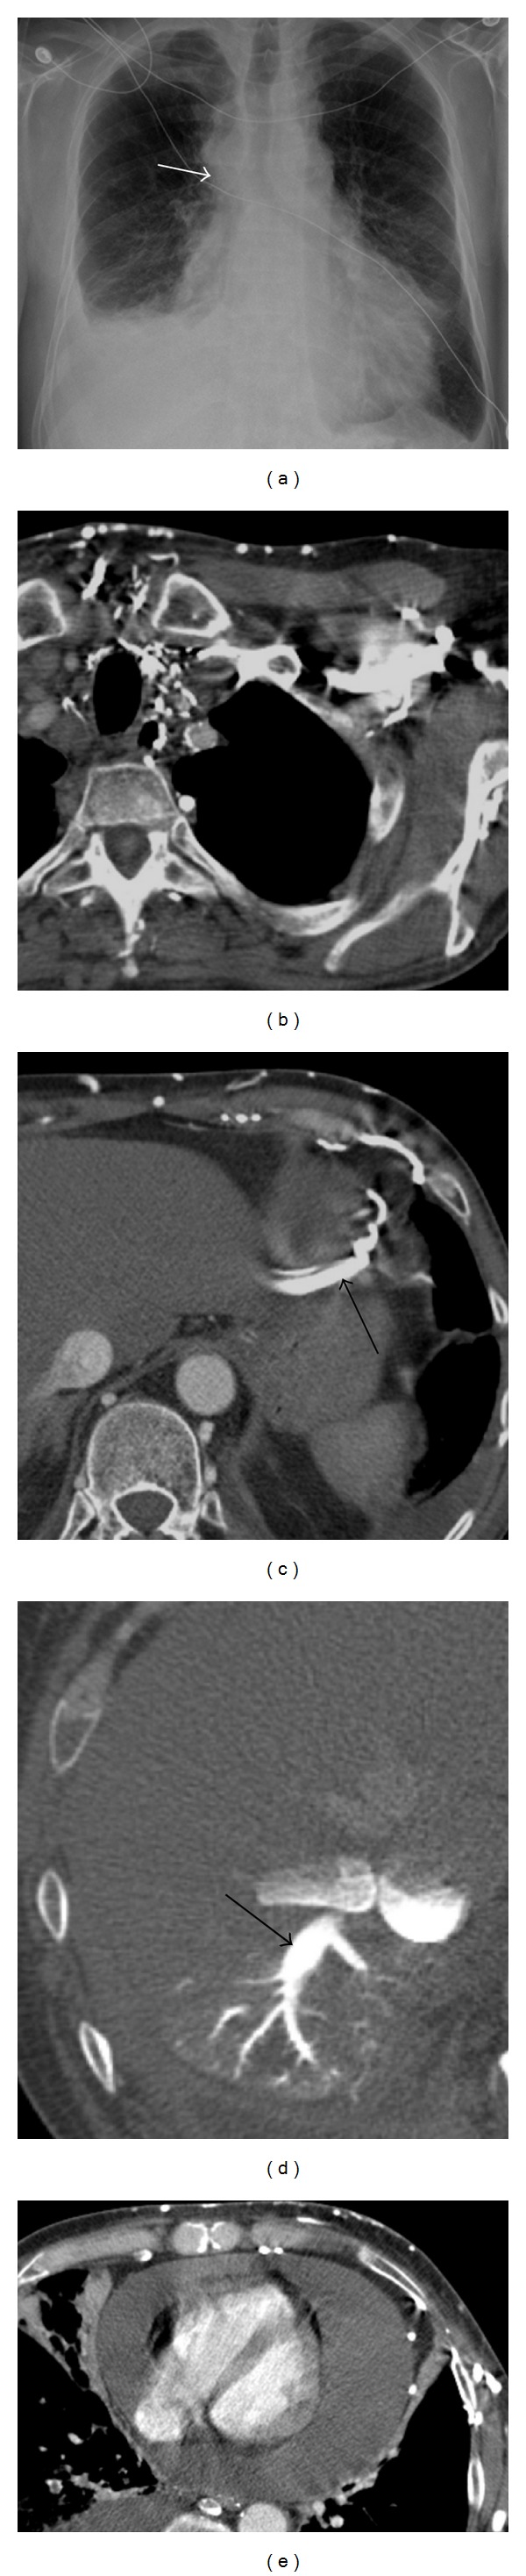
A 55-year-old previously healthy male with dyspnea. (a) Chest radiograph shows an enlarged cardiac silhouette, a right pleural effusion, and dilated azygos vein (arrow), (b) CECT demonstrates extensive venous collaterals around the heart, including prominent filling of (b) subcutaneous, (c) inferior phrenic, and (d) hepatic veins. (e) CECT shows a large pericardial effusion. The interventricular septum is flattened. The constellation of effusion, flat septum, and impaired venous return (b–d) is consistent with tamponade physiology. The patient's symptoms and blood pressure improved after pericardiocentesis. Fluid cytology was positive for malignant cells. He was later diagnosed with nonsmall cell lung cancer.
